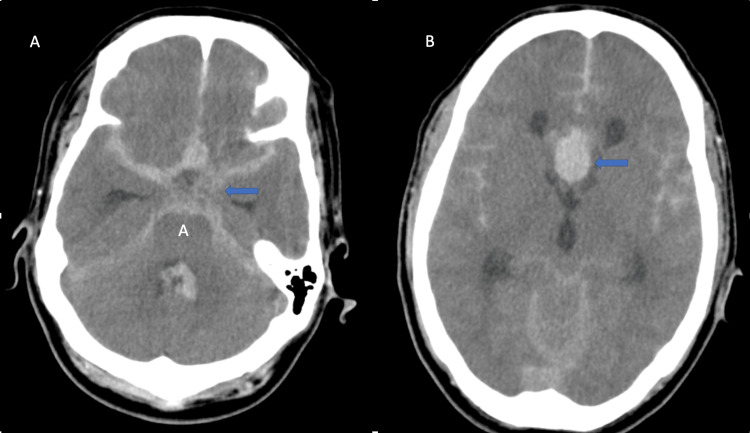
Plain CT of the brain (axial sections) showing diffuse, thick subarachnoid hemorrhage involving the perimesencephalic and suprasellar cisterns (blue arrow in A) and bilateral Sylvian cisterns, with the densest collection in the interhemispheric fissure (blue arrow in B). Associated intraventricular hemorrhage and ventriculomegaly are noted, corresponding to a modified Fisher Grade 4
